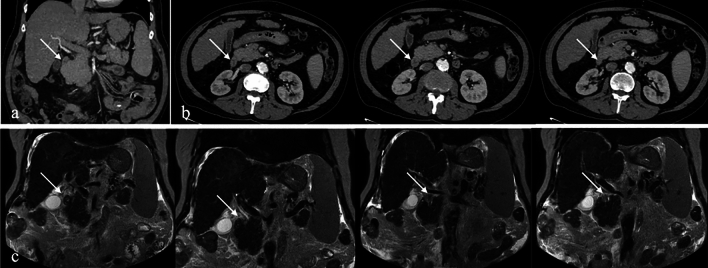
IV contrast-enhanced a. Coronal and b. Axial CT images. c. Coronal T2-weighted MRI image of a 58-year-old male patient with chronic liver disease. The images demonstrate annular pancreatic tissue encircling the second (D2) segment of the duodenum (arrows), consistent with annular pancreas
